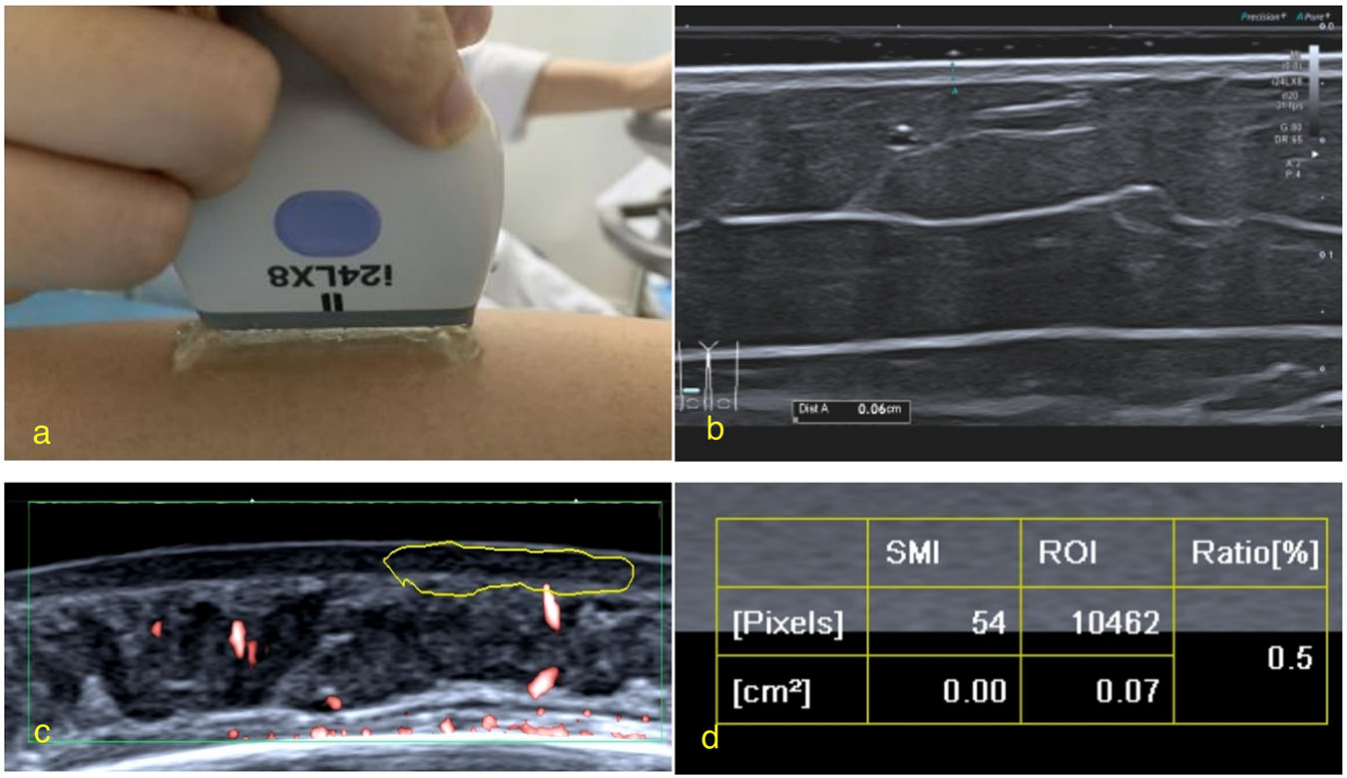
Ultrasonic examination and measurement. a The ultrasonic probe was placed vertically without exerting pressure or direct skin contact. b Measurement map of skin layer thickness on the right thigh. c Vascular index (VI) measurement image of microvascular blood flow in the skin layer for normal skin on the left forehead. d The manually measured pixel value in the region of interest (ROI) was 10462, and the VI was 0.5%
